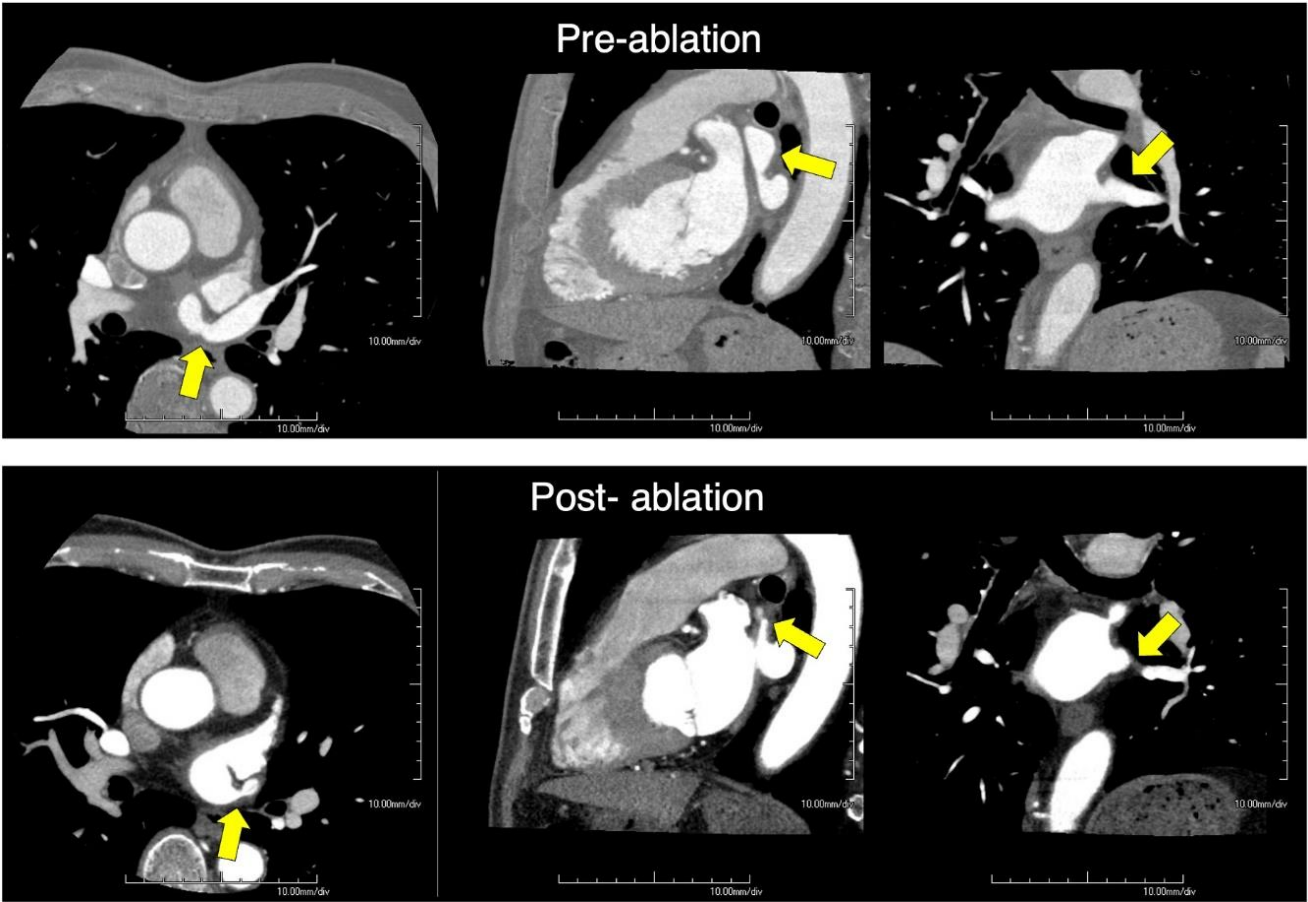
Case 2. Pre-and Post-Ablation CE-CT images
